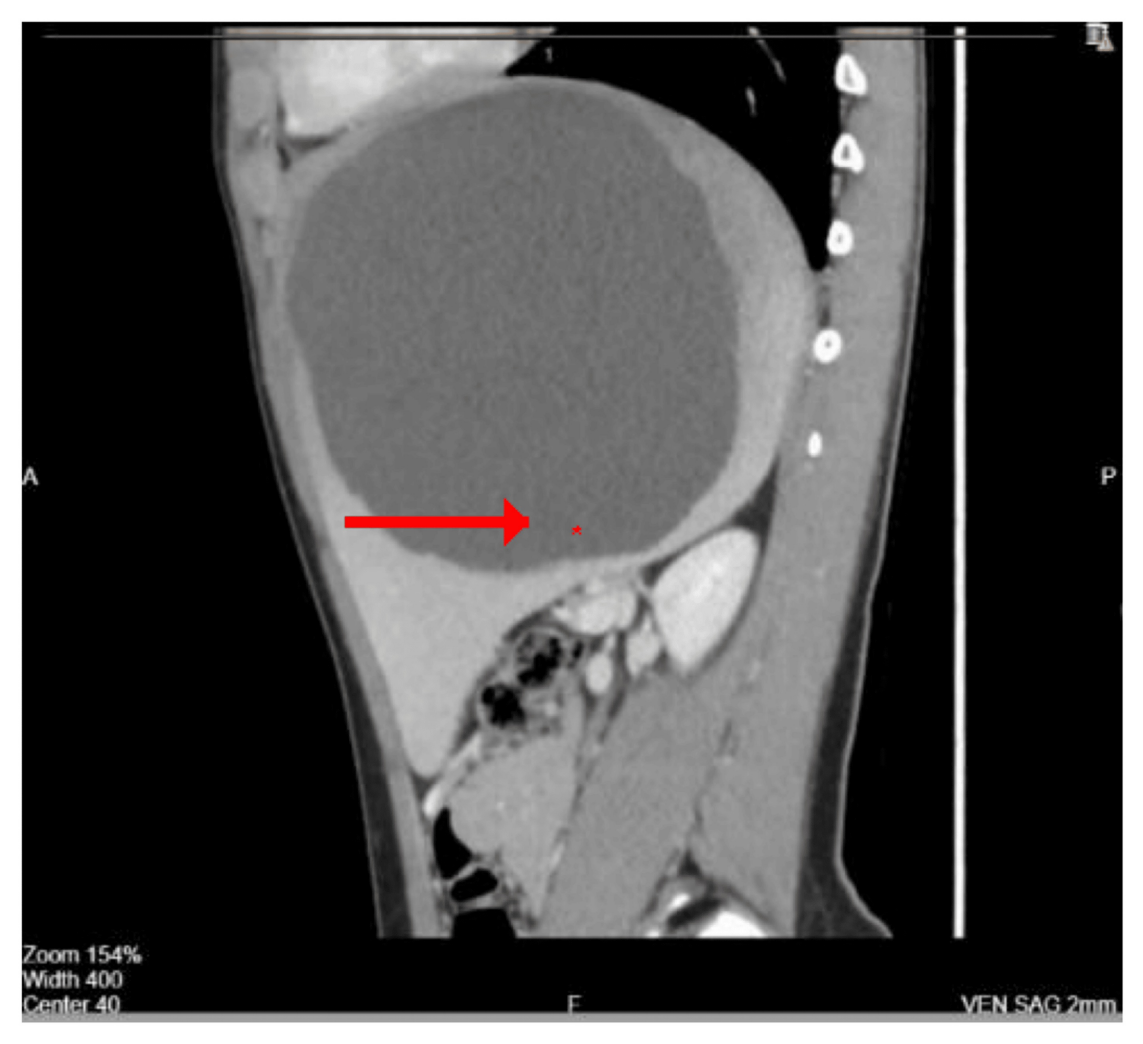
Sagittal CT scan demonstrating the cyst dimensions and its relation to surrounding organs. Sagittal contrast-enhanced CT view revealing the vertical extent of the splenic cyst and its close relationship to the diaphragm and stomach (asterisk marks cyst cavity).
